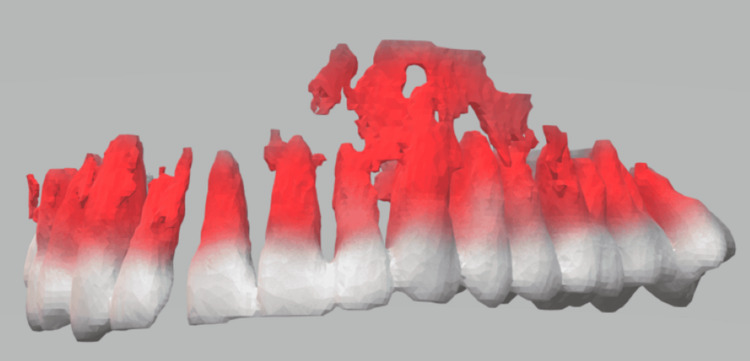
Digital intraoral scan obtained with a 3Shape TRIOS (3Shape, Copenhagen, Denmark) scanner for occlusal reconstruction and 3D planning. Surface-rendered intraoral scan of the maxillary dentition showing the teeth (white) and gingival/soft-tissue surfaces (red). The scan was used to generate a digital dental model for preoperative occlusal registration and integration with CT-based 3D modeling when conventional impressions were not feasible. CT, computed tomography
